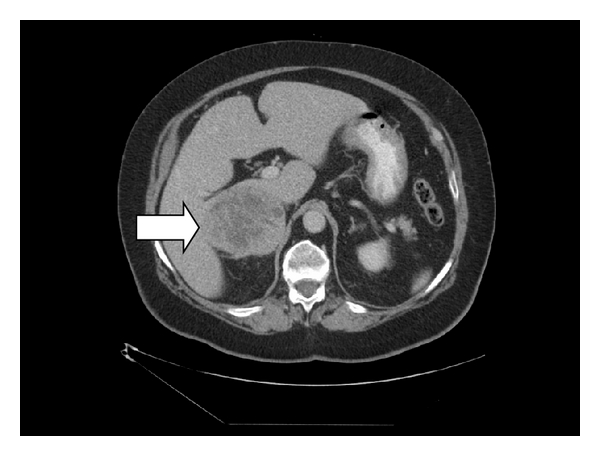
CT of the abdomen showing an adrenal mass adherent to the IVC.
